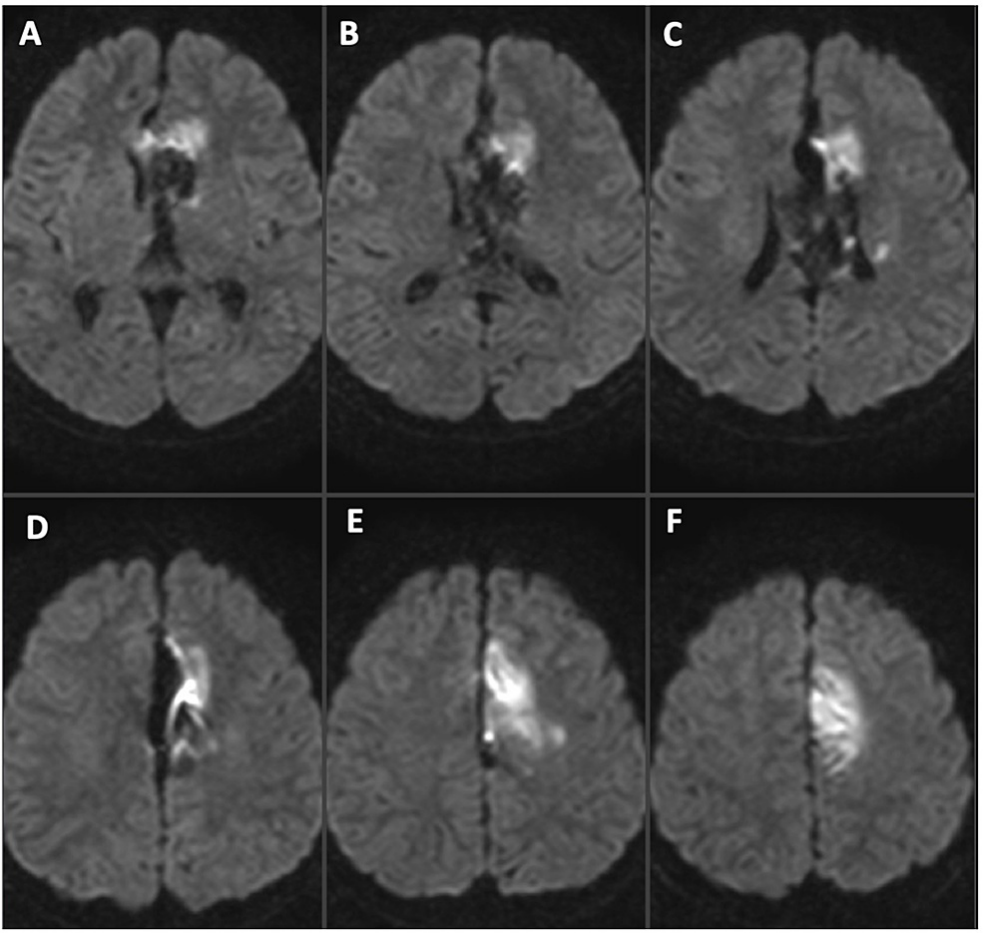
Post-embolization MRI (DWI) Post-embolization diffusion-weighted imaging (DWI) demonstrating infarction of Lt. ACA branch feeding area. Slice is from thalamus (A) level to leg area of the primary motor cortex (F). Infarct area was supplementary motor cortex to primary motor cortex of the leg area (E)(F), cingulate gyrus (D), and corpus callosum (A)(B)(C).
